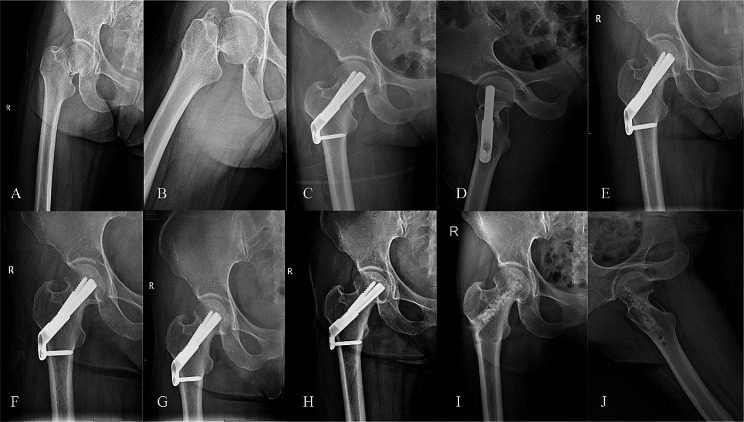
Radiographic images of a typical clinical case: X-ray during preoperative examination (A, B); Placement of FNS after closed reduction (C, D); X-ray at 3 months postoperatively (E); X-ray at 6 months postoperatively (F); X-ray at 12 months postoperatively (G); X-ray at 18 months postoperatively (H); Bone grafting performed after removal of internal fixation (I,J)
